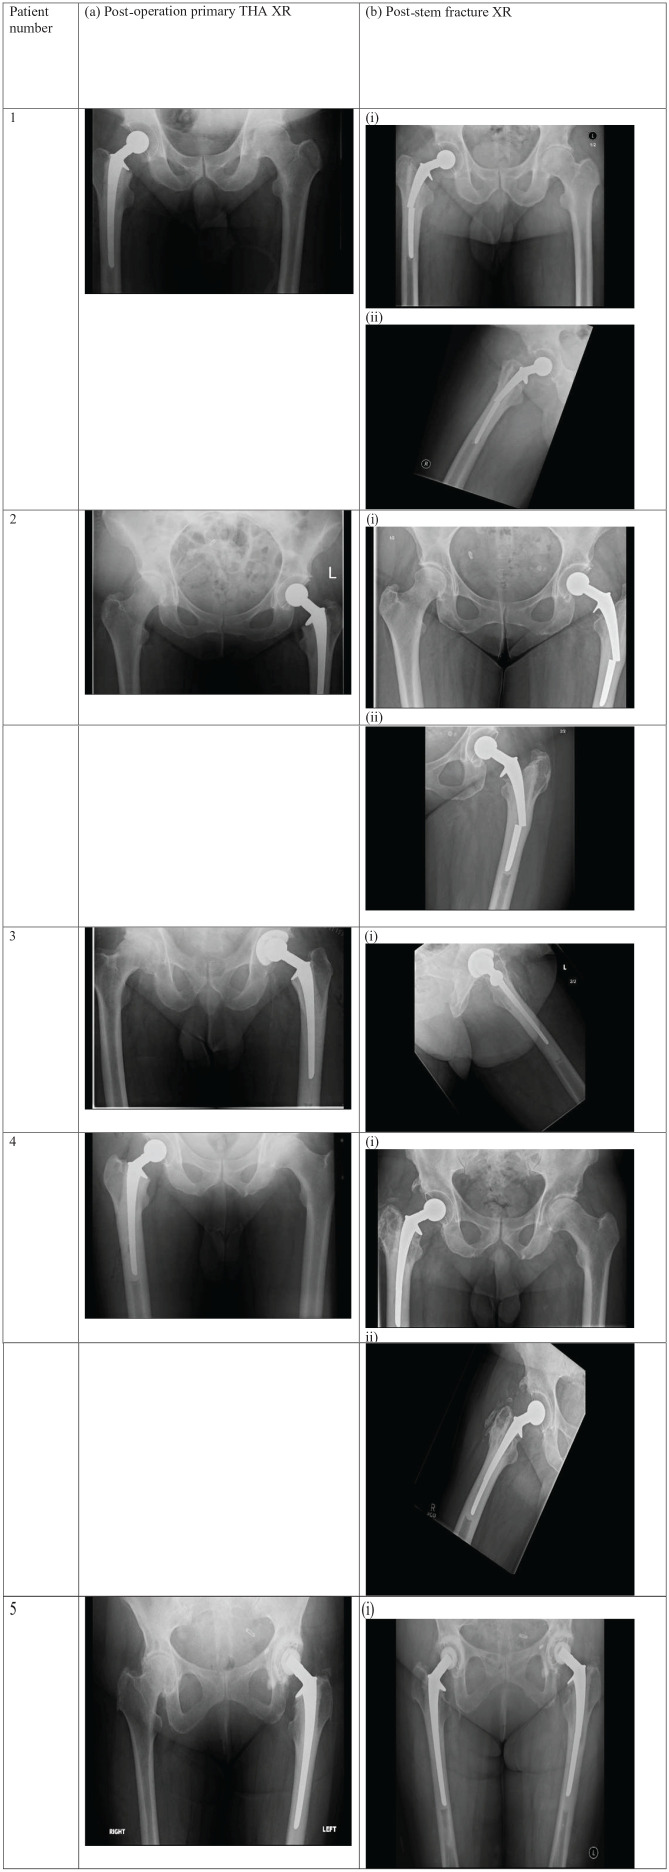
X-ray images following primary THA and subsequent stem fracture. *Note.* X-rays following primary THA (a) and femoral stem fracture (b) (Patients 1–5). (i) Anteroposterior view. (ii) Lateral view congruent with the fractured stem (see Table 2).THA = total hip arthroplasty.
